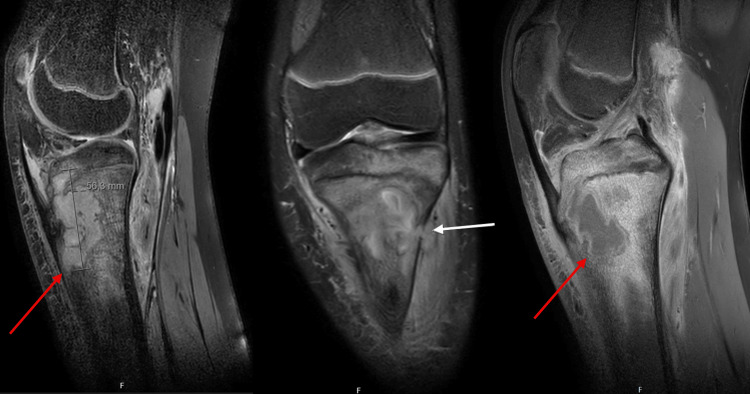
MRI MRI of the left knee showing a large intraosseous abscess cavity in the proximal tibia measuring approximately 56 mm (red arrow), with surrounding bone marrow edema and cortical breach (white arrow). The imaging features are consistent with subacute osteomyelitis (Brodie’s abscess). The T1-weighted image demonstrates a central hypointense cavity surrounded by a hyperintense granulation rim (“penumbra sign”) with adjacent marrow edema.
